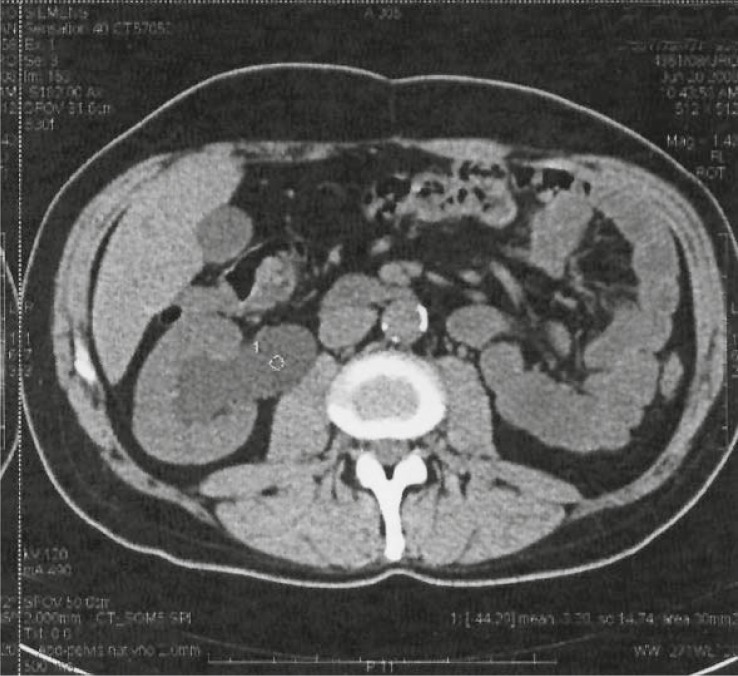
Multislice computed tomography (MSCT) - right hydronephrosis.
